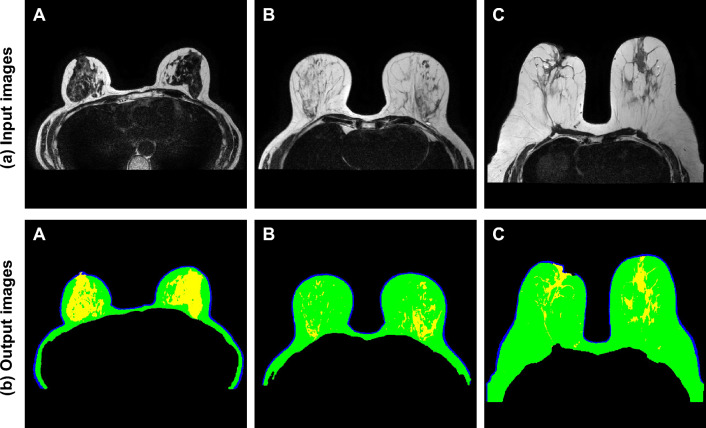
Original MRI images (top) and breast tissue images segmented through the U-Net based on Haar wavelet pooling (bottom) for three female patients.
